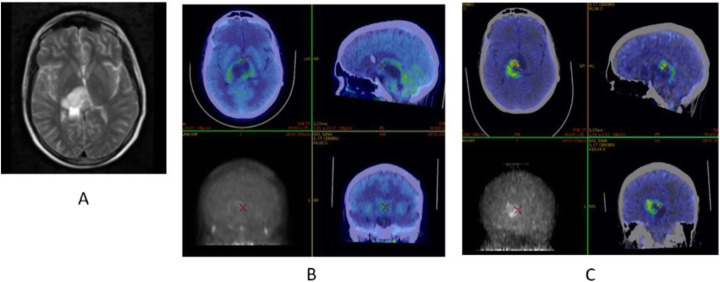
^18^F-FMISO, characterisation of a brain lesion and comparison to ^11^C-methionine. *Clinical history*: 15 y.o. boy. High-grade glioblastoma, sub-totally removed and treated with radiotherapy. MRI: persistence of expansive lesion in thalamic area (a). *PET/CT findings*: ^11^C-methionine shows peripheral uptake in the right thalamus tumour, probably related to residual viable tumour or relapse despite its low intensity (b). ^18^F-FMISO PET/CT showed hypoxic area in previously methionine uptake described lesion (c), suggesting a relapse
